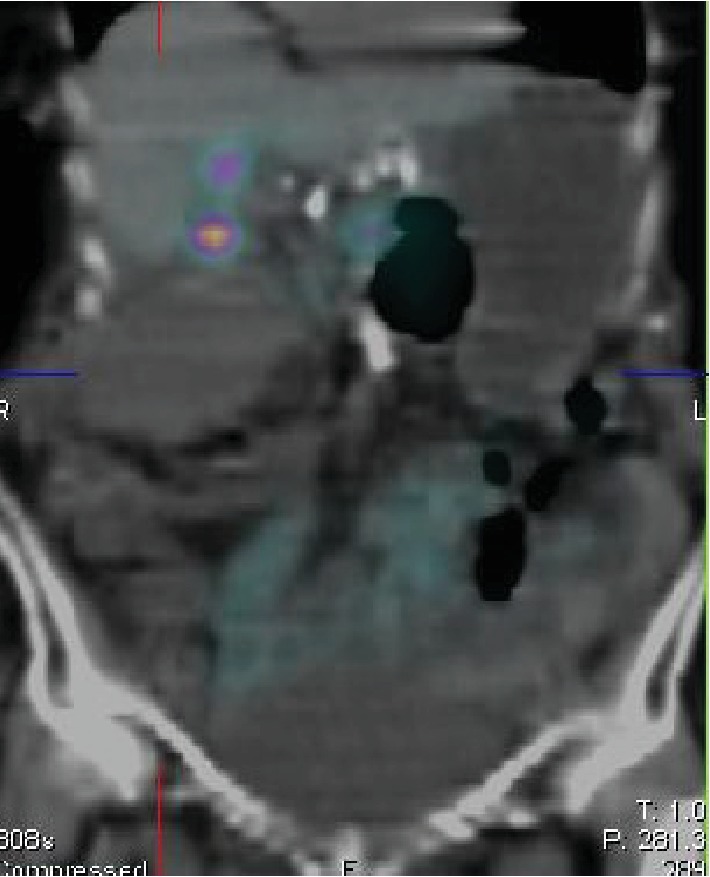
SPECT-CT combining HIDA and CT scans demonstrating discrepancy in gallbladder imaging.
